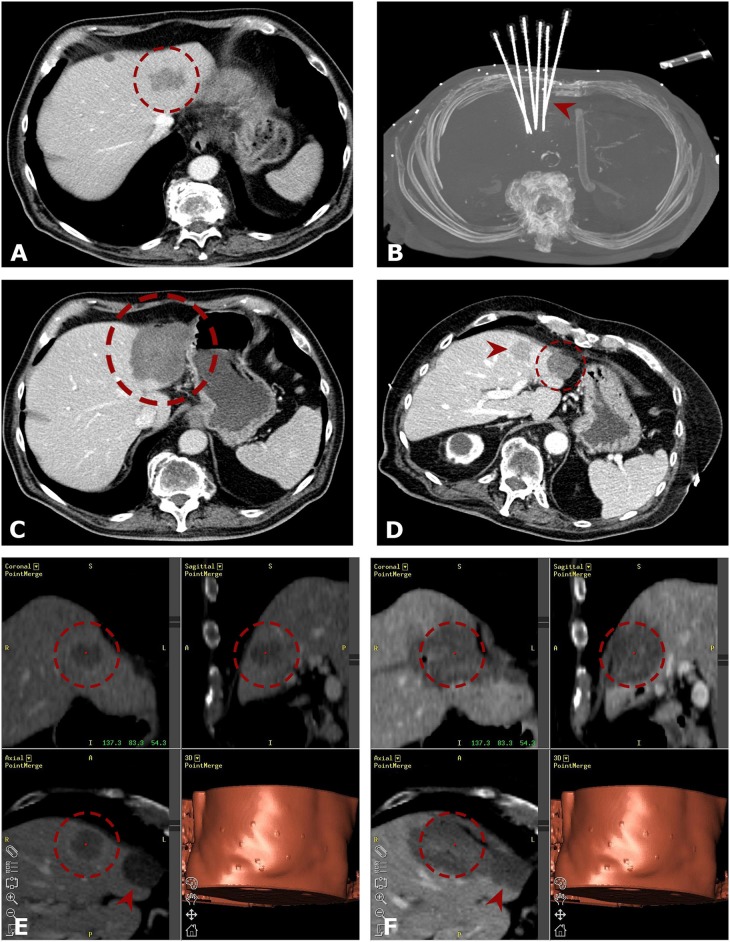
Case of a 80-years old male with a singular 4.0 cm HCC in segment II. (A) Portal venous phase initial CT-scan with a hypo-enhancing nodule in segment II (*red dashed circle*). The enhancement of the HCC is partly atypical (slight arterial flushing). (B) MIP of the native control CT with 6 needles in place (*red arrowhead*). (C) Axial portal venous ce-enhanced CT after 3 months showing complete coagulation zone (*red dashed circle*). (D) The *red dashed circle* is marking the progressively shrinking coagulation zone at 48 months. New distant tumor recurrence is highlighted by the *red arrowhead*. (E,F) Repeated SRFA of the distant tumor recurrence (*red dashed circle*). Fused images from the navigation system with 3D views from planning CT (E) and final control CT (F) with complete necrosis including a sufficient ablation margin. The *red arrowhead* is marking the initial ablation zone.
